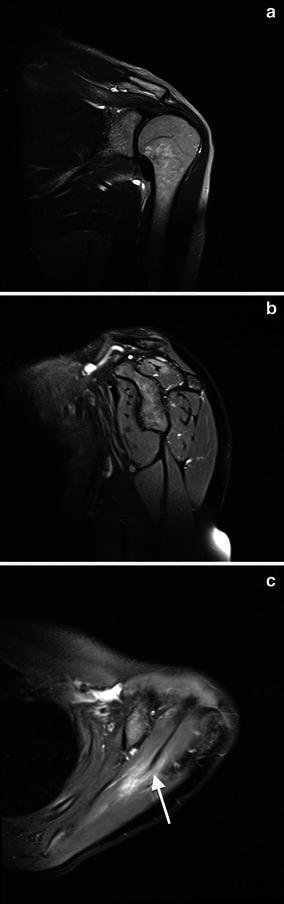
Magnetic resonance imaging performed 60 days after trauma. a T1 short-tau inversion recovery coronal and b proton-density fat saturated sagittal views show reduction of the intra- and perimuscular edema, but a persistent small intramuscular area of high signal compatible with edema remained in the site of the lesion. c Proton-density fat-saturated axial view shows a more homogeneous and stretched intramuscular septum (*arrow*)
